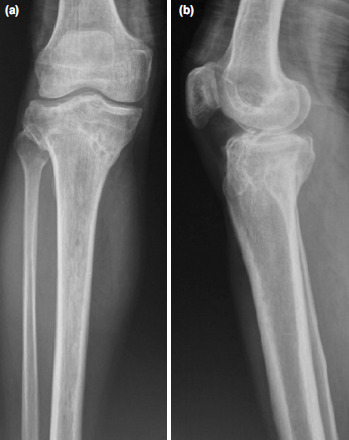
Case No.7, radiographs of knee at final follow up showing fracture union and well-maintained alignment. (a) Antero-posterior view. (b) Lateral view.
